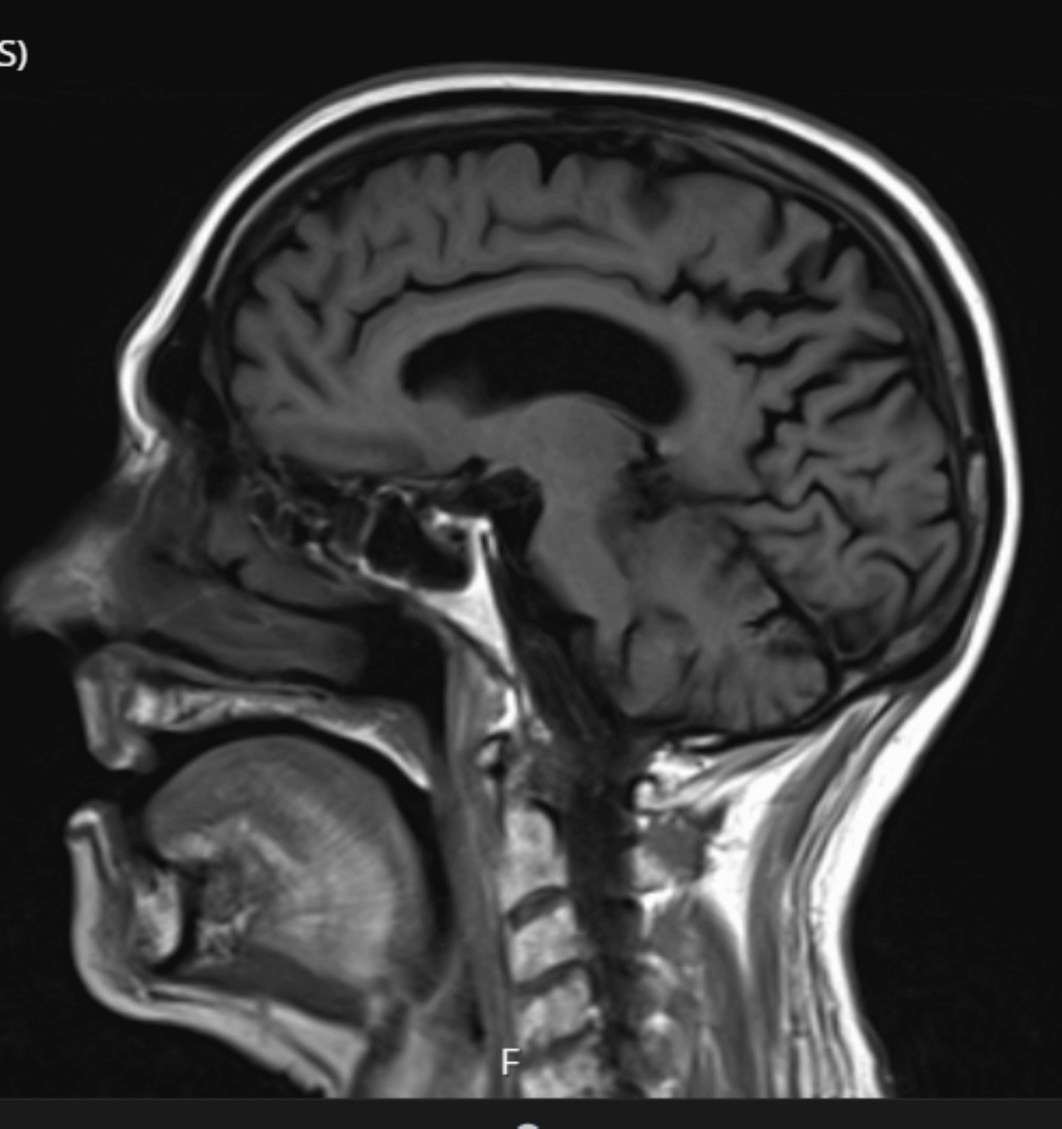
Sagittal T1-weighted MRI demonstrating global age-related involutional changes Sagittal T1-weighted MRI demonstrating cortical thinning and mild ventricular prominence consistent with global involutional changes.
